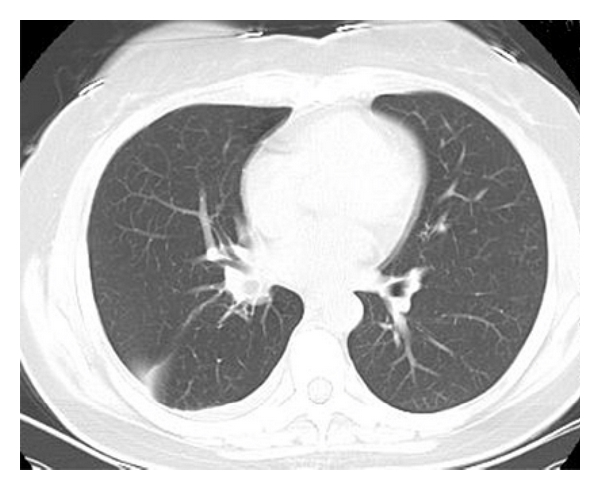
Axial CT showed the occlusion of right inferior basal branch.
